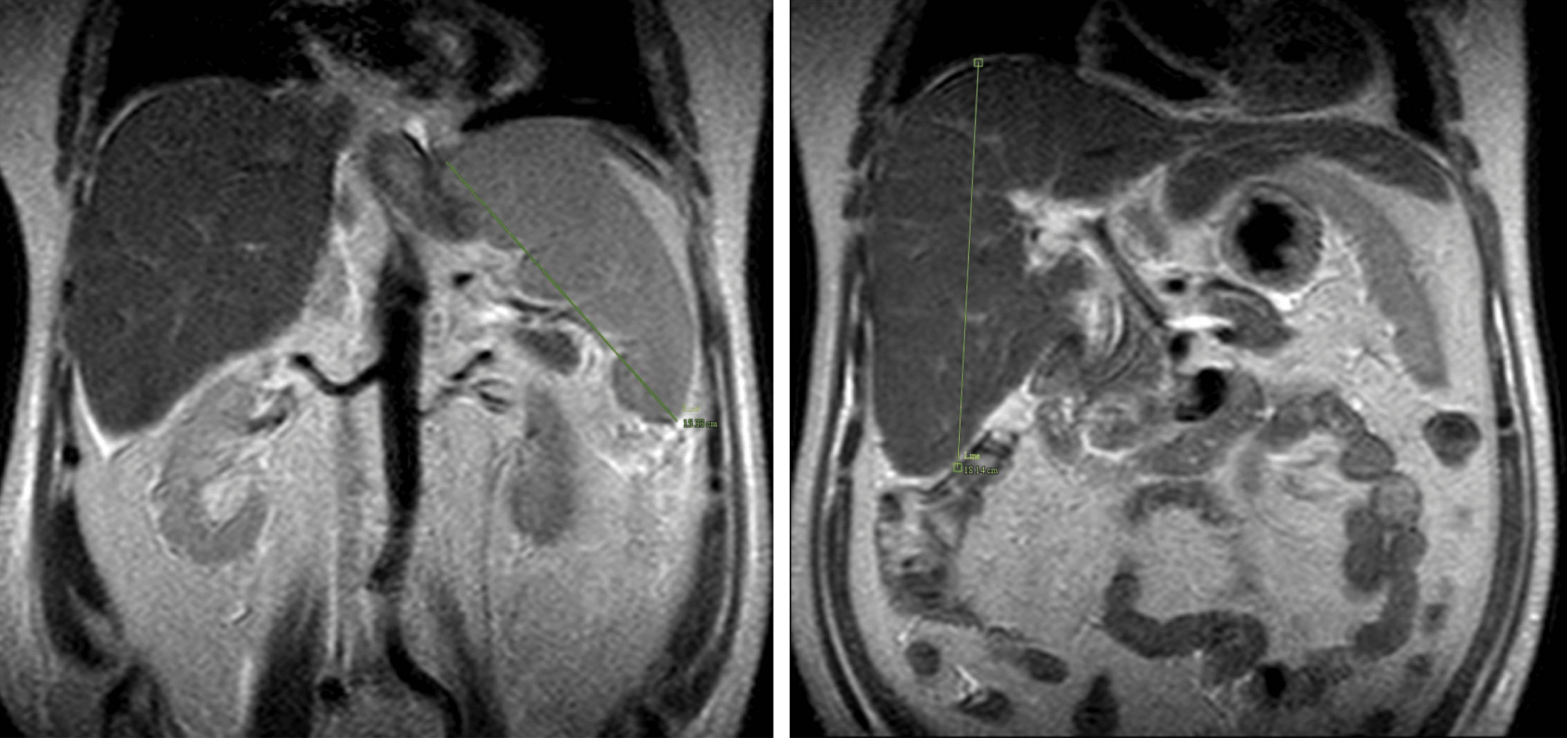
MRI of the abdomen (plain image) MRI of the abdomen (plain image) demonstrating hepatomegaly on the right, with a liver span of 18.14 cm, and splenomegaly on the left, with a splenic span of 15.33 cm.
